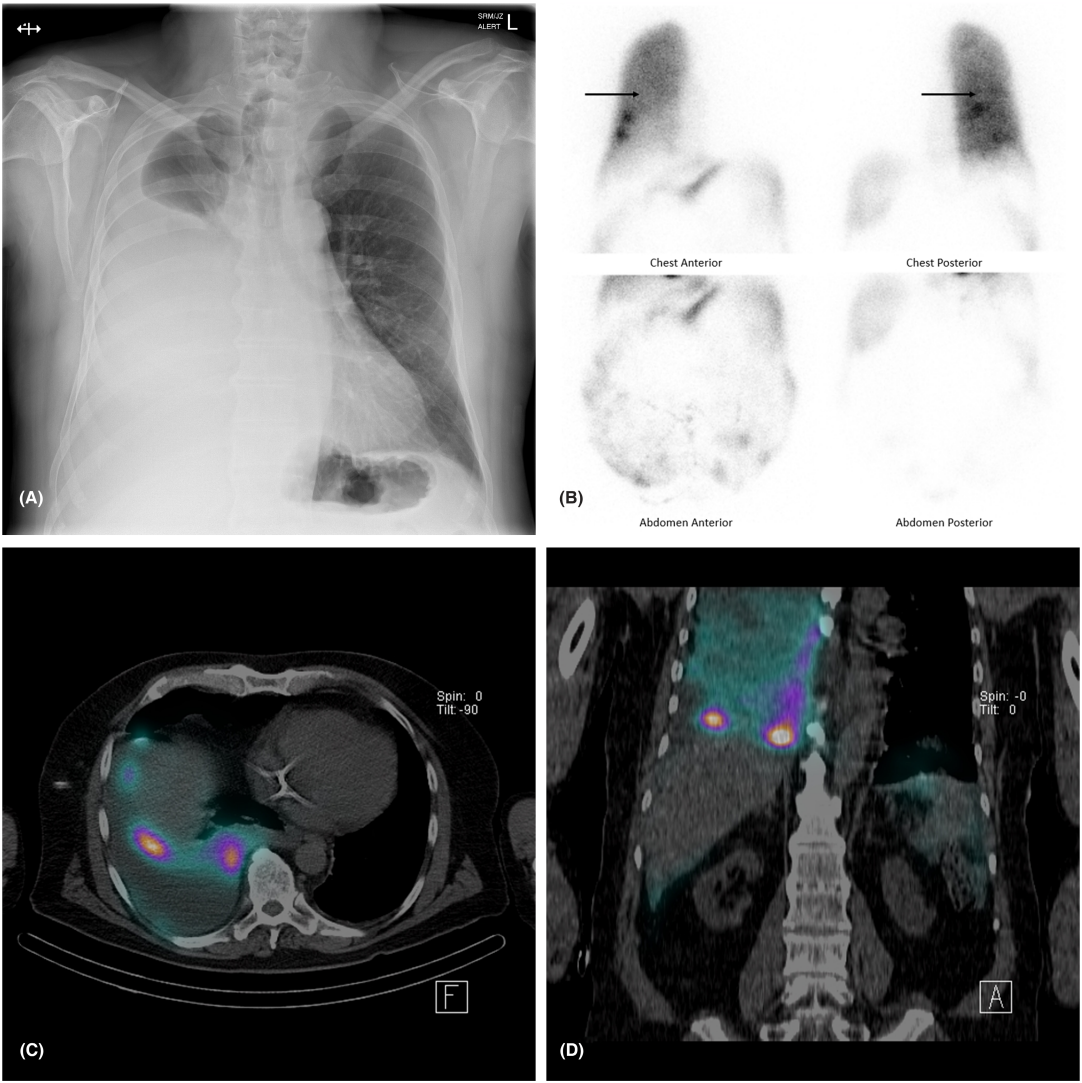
(A) Posterior–anterior chest radiograph demonstrating a large right‐sided pleural effusion. (B) Peritoneal scintigraphy planar images at 1 h following administration of dialysate fluid containing 111 MBq Tc‐99m macroaggregated albumin. Pleuroperitoneal communication is observed with radiotracer activity diffusely within the peritoneal cavity and right hemithorax (arrows). (C, D) Axial and coronal sections of SPECT–CT display right‐sided pleural effusion accumulated with radiotracer and multiple foci along the right hemidiaphragm. A clear fistula was not identifiable. Tunneled hemodialysis catheter in situ.
